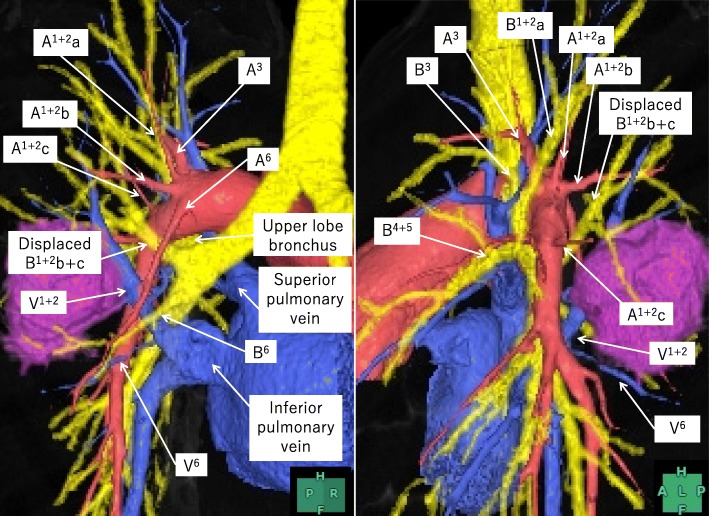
Three-dimensional computed tomography with multiplanar reconstruction. The displaced B^1+2^b+c passes to the dorsal side of the left main pulmonary artery. The branch of A^6^ arises from the left main pulmonary artery at the level of the branches of A^3^ and A^1+2^ and passes to the dorsal side of the displaced B^1+2^b+c. The branch of V^1+2^ passes between B^6^ and the bronchus to the basal segment and joins V^6^ at the dorsal side of the pulmonary hilum
